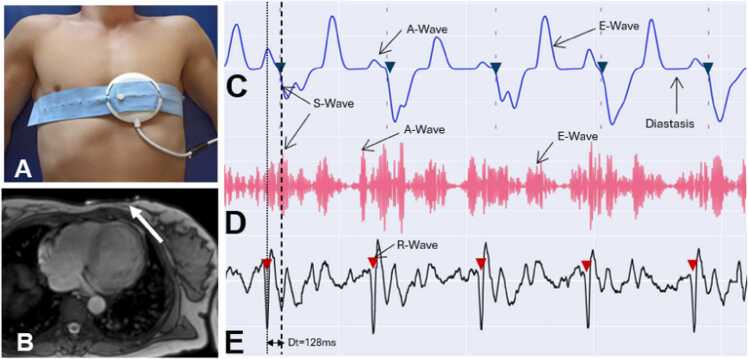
(A) Typical position of the Doppler ultrasound (DUS) transducer fixed with a belt. (B) Position of the transducer (white arrow) relative to the heart position. (C) The filtered DUS signal of patient 12. (D) The demodulated Doppler signal filtered between 60 and 100 Hz. The used S-wave for DUS triggering is marked in blue, and the R-wave is marked in red. The trigger occurs in average 128 ms after the R-wave. It can be seen that part of the delay is due to the filter delay time of the filtered signal in (D) that results in the signal shown in (C). Also indicated are the A wave which occurs during atrial contraction, the E-wave during isovolumetric contraction and the diastasis as the resting period
